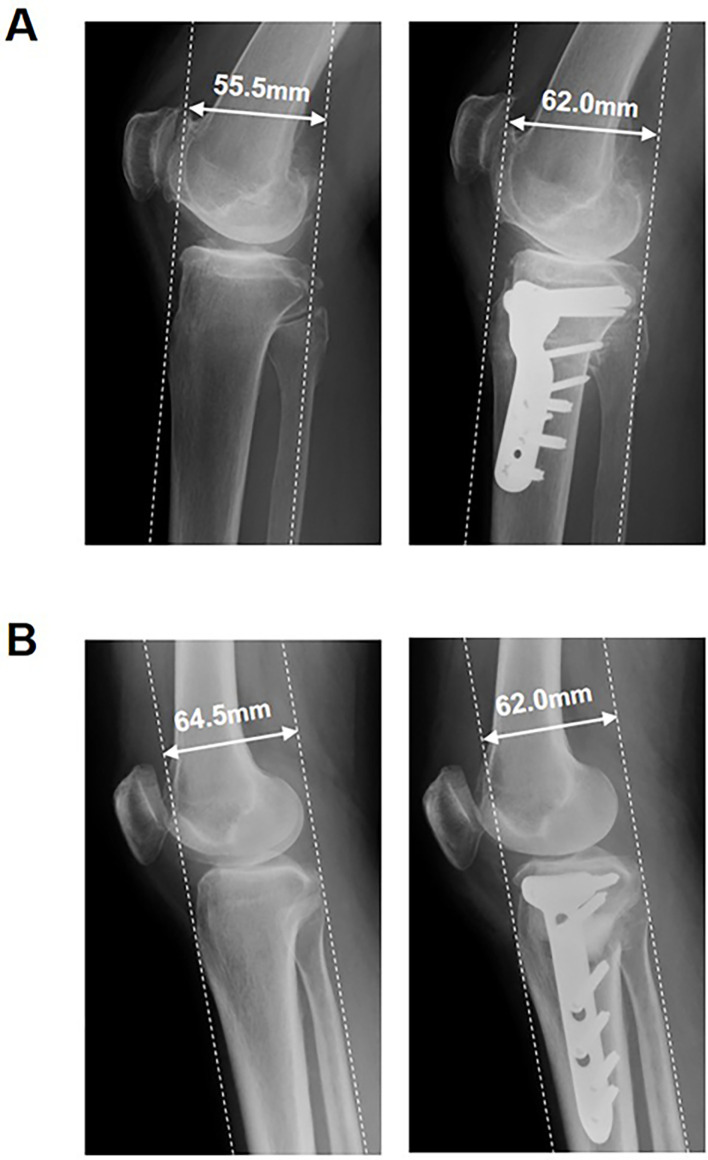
Pre- and postoperative radiographs from one patient each who underwent closed wedge high tibial osteotomy (A) and opening wedge high tibial osteotomy (B). (A) The distance between the anterior border of the tibial tuberosity and the posterior border of the medial tibial condyle is 55.5 mm preoperatively (left) and 62.0 mm postoperatively (right), indicating an increase of 6.5 mm. (B) The distance between the anterior border of the tibial tuberosity and the posterior border of the medial tibial condyle is 64.5 mm preoperatively (left) and 62.0 mm postoperatively (right), indicating a decrease of 2.5 mm.
